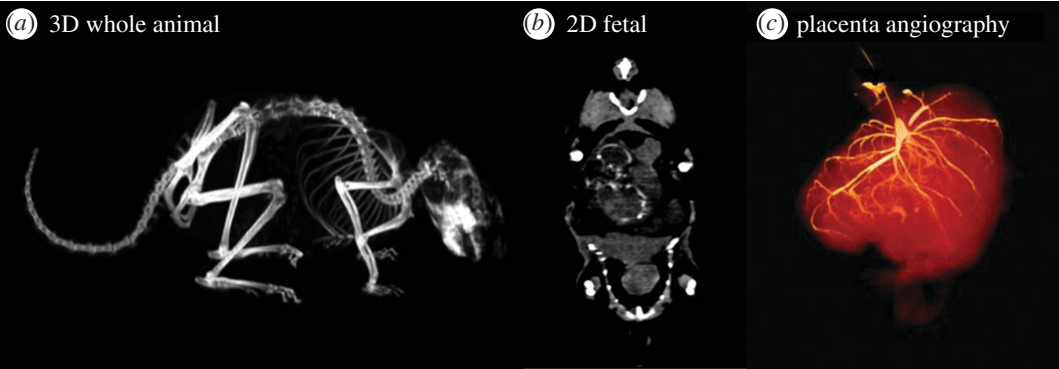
CT scan on whole chinchilla (*a*,*b*), demonstrating the low-radiopaque fetus, and CT-angiography of chinchilla placenta (*c*), showing a volume of the full placenta on 4000 mm^3^ and the fetal vasculature on 66 mm^3^ (more than 400 HU) and 193 mm^3^ (more than 250 HU).
